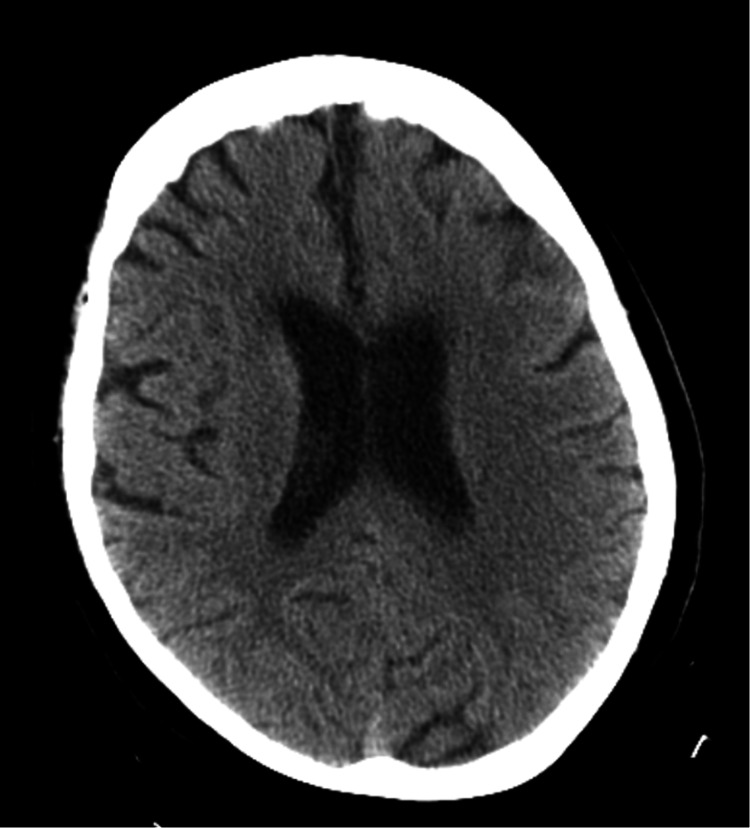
Non-contrast computed tomography of the head at admission negative for acute intracranial bleed, infarct, or cerebral edema
